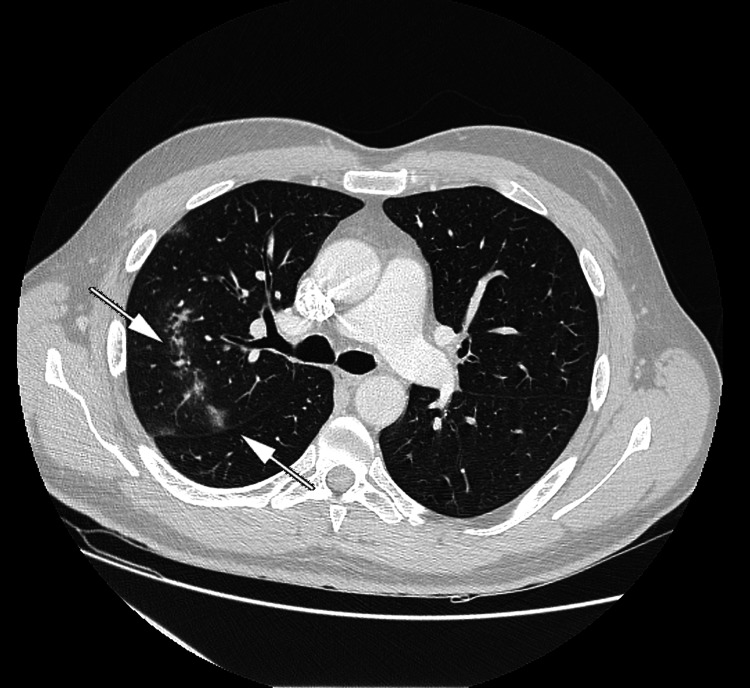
Thoracic CT scan Slide of a thoracic CT scan revealing linear-shaped ground glass opacities in a transversal plane in the upper right lobe (arrows), which resembled round-shaped opacities in the frontal plane of a plain chest X-ray
